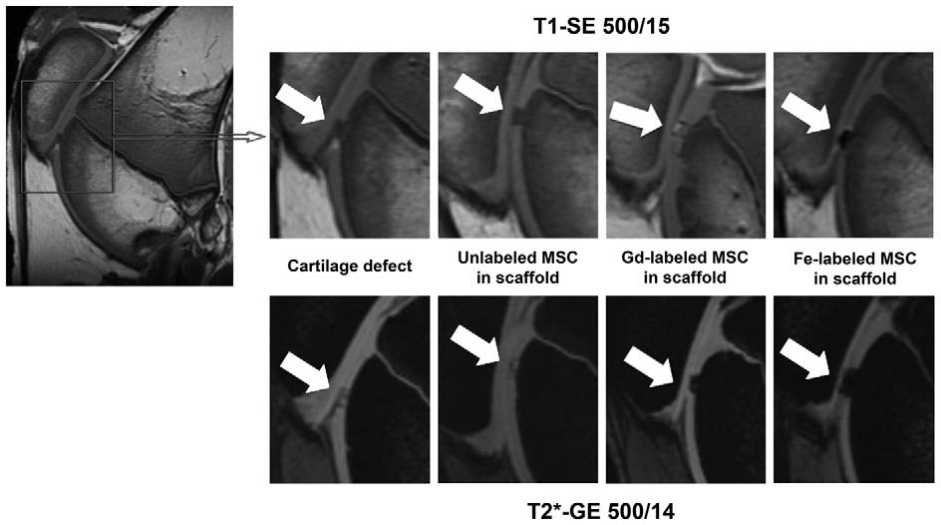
Representative MR images of matrix associated stem cell implants (MASI). Sagittal T1-SE sequences (upper row) and T2* GE sequences (lower row) of cartilage defect, unlabeled MSC in scaffold, Gd-labeled MSC in scaffold, Fe-labeled MSC in scaffold in the femoral condyles (arrows).
